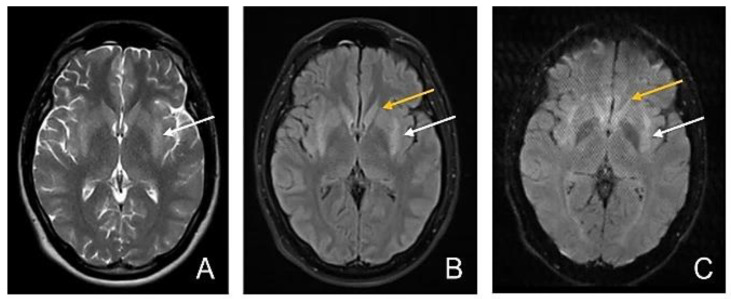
Third MRI—D11. (A) Axial T2-wi sequence: persistent high T2 signal intensity in posterior 2/3 of the putamen; (B) Axial FLAIR image: Symmetrical hyperintensity of the posterior 2/3 of the putamen (white arrow) and normal signal intensity of the caudate nucleus (yellow arrow); (C) Axial DWI: persistent symmetrical moderate restricted diffusion in the posterior 2/3 of the putamen (white arrow) and no restricted diffusion in the caudate nucleus (yellow arrow).
